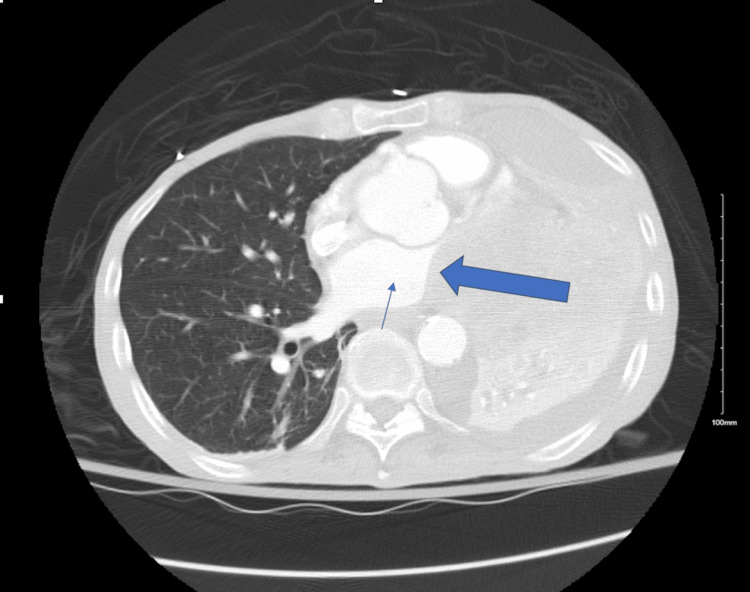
CT chest with contrast Shows mass compressing and invading the left atrium (big blue arrow) and left atrium (small blue arrow).
